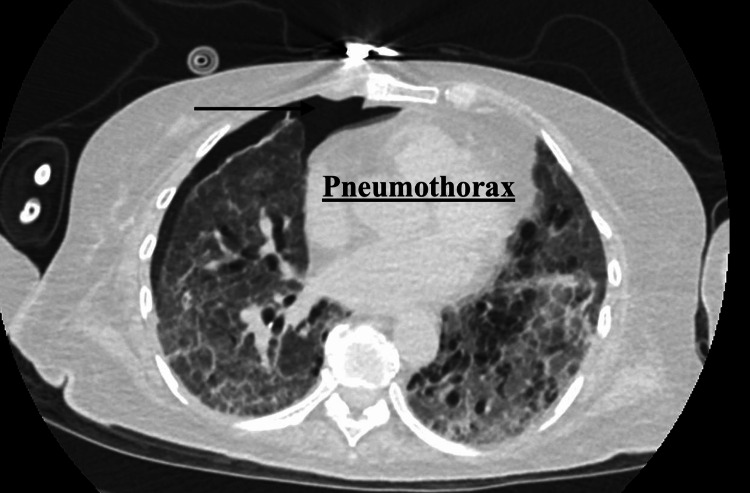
CT scan imaging: pneumothorax A pneumothorax is defined as the presence of air within the pleural space, which causes partial or complete collapse of the underlying lung. This occurs when there is a disruption of the visceral or parietal pleura due to trauma, lung disease, or spontaneous rupture of alveoli or blebs. For our patient, this was seen secondary to her open lung biopsy with chest tube placement and subsequent removal. CT, computed tomography
